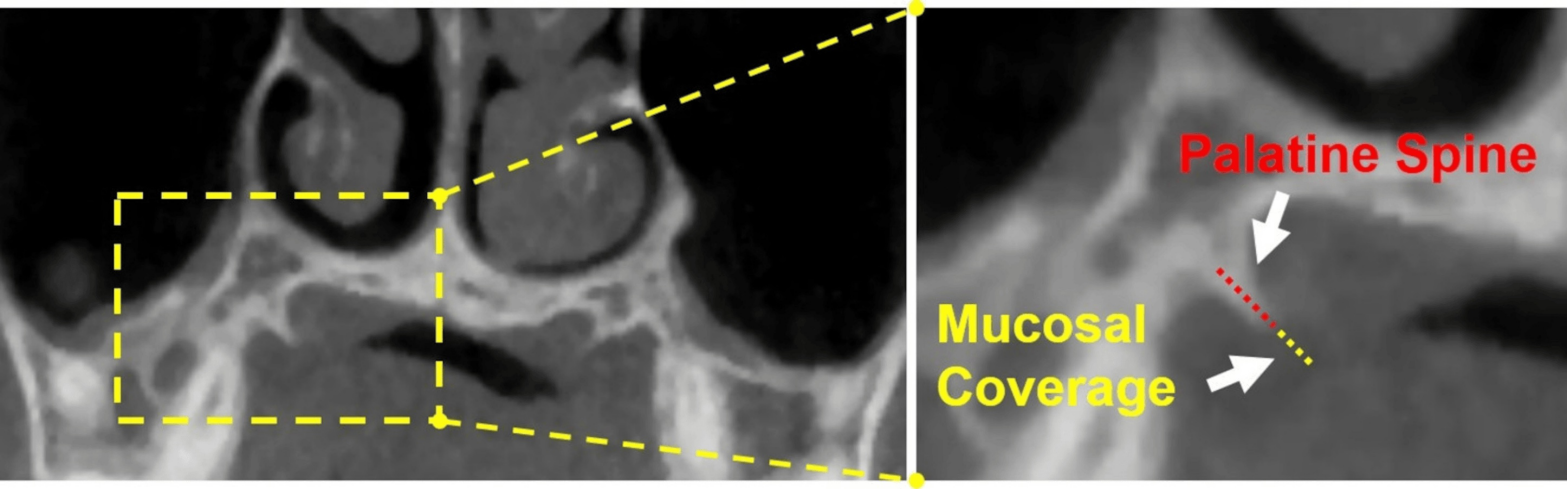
Location of PS and its mucosal coverage Left image shows a CT coronal image through the posterior hard palate demonstrating a right middle PS (within inset). Right image presents inset details of measurements made for the length of PS (red dotted line) and of its mucosal coverage (yellow dotted line). PS: Palatine spine
